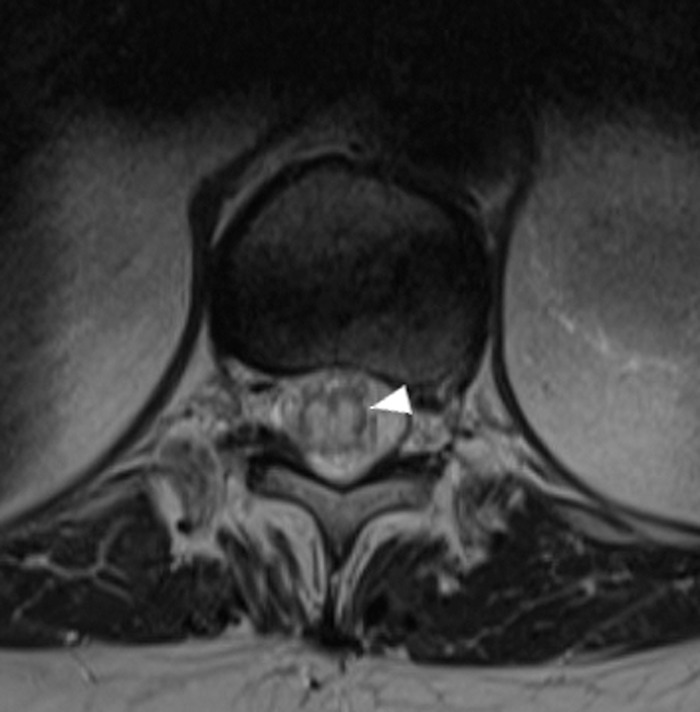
Axial T2-weighted image through the level of the mid-thoracic spinal cord demonstrates long segment central cord hyperintensity and expansion in the lower thoracic cord (arrowhead).
